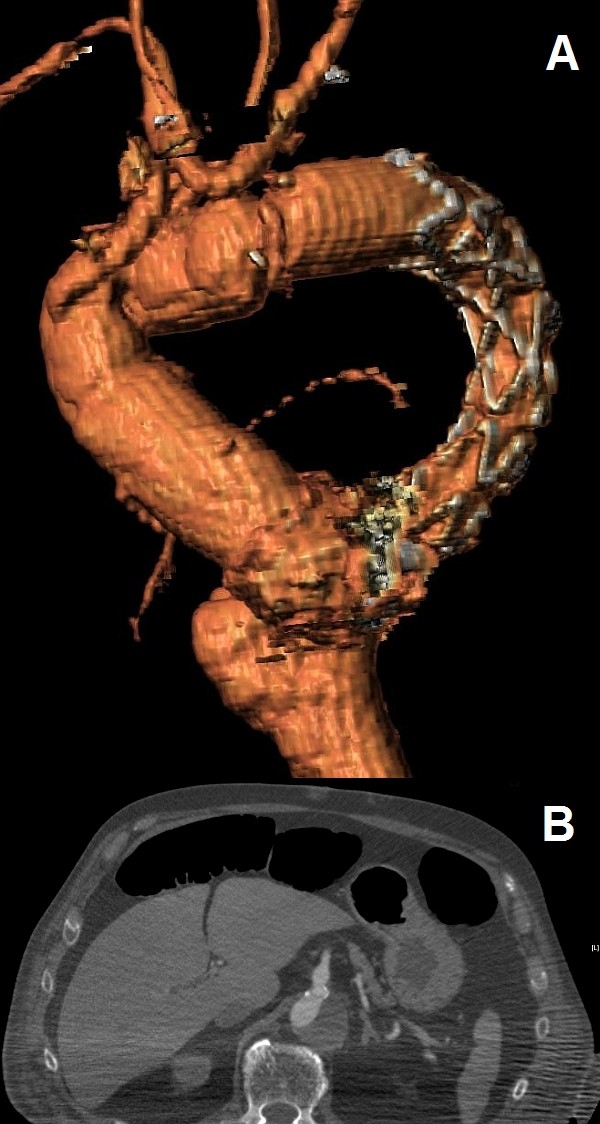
Postoperative images of the aorta . (a.) 3D CT reconstruction image of the thoracic aorta showing complete replacement of ascending aorta and aortic arch, the FET in the descending thoracic aorta and the saphenous vein grafts originating from the ascending aorta. (b.) Axial CT image demonstrating good flow in the celiac trunk.
